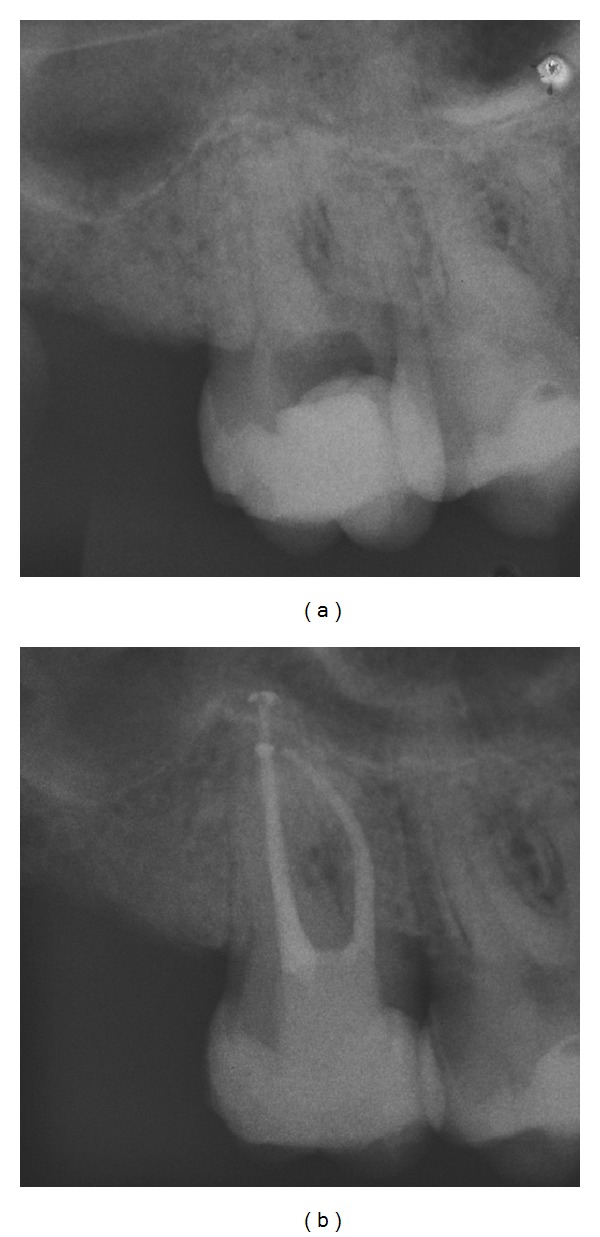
(a) Initial radiograph showing constricted canals mainly in the buccal roots. (b) Final radiograph.
